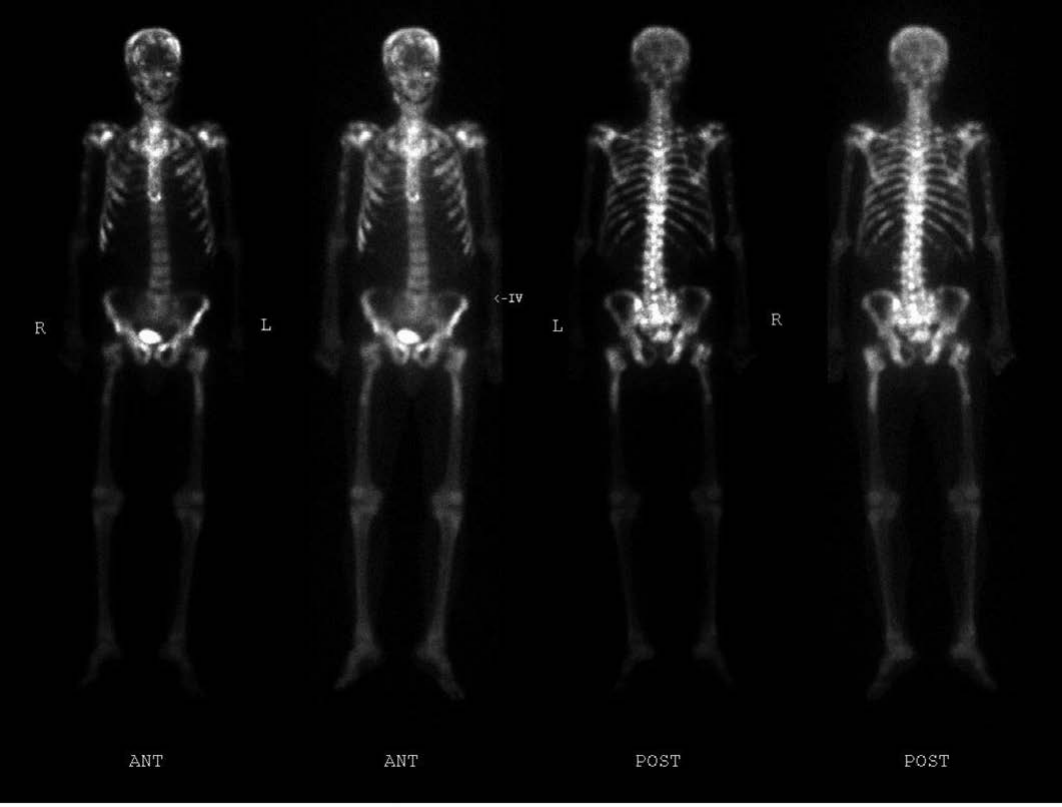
Bone scintigraph showing extensive bone metastases.
